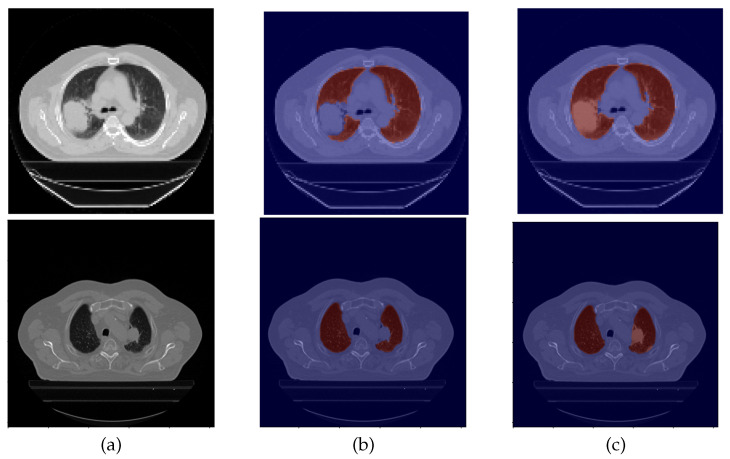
Example of a data correction. From left to right: (a) CT original image; (b) the original lung mask, which excludes the nodule; (c) and the corrected lung mask, which includes the nodule.
